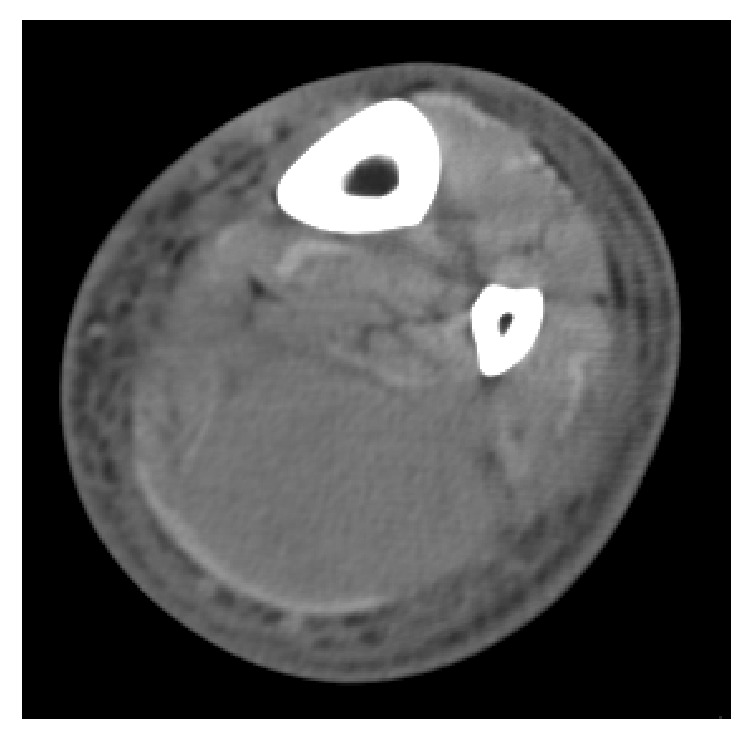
Axial contrast enhanced CT image of the 55-year-old man from case 2 showing diffuse enlargement of the soleus muscle which is homogenously iso- to slightly hypodense relative to adjacent muscle. Note the skin thickening and subcutaneous fat stranding, which can also be seen in lymphoma.
